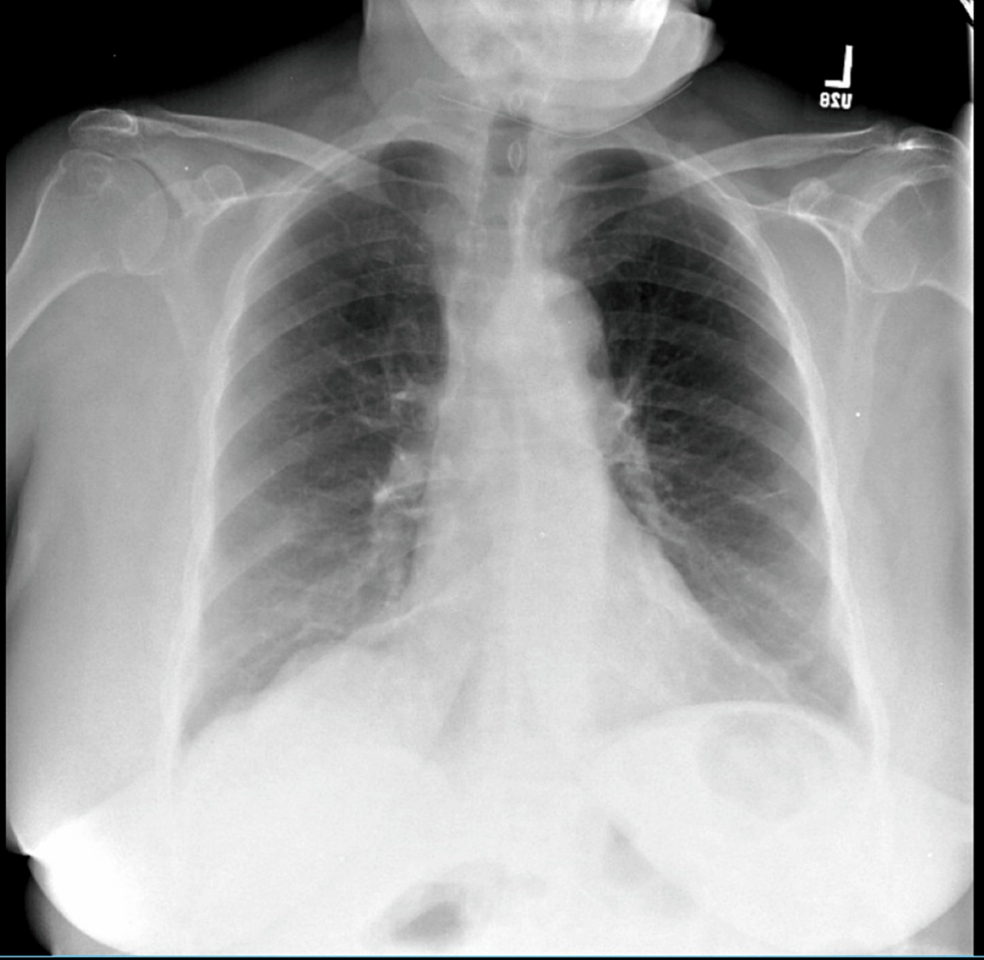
Chest X-ray showing mild atelectasis in both lung bases
